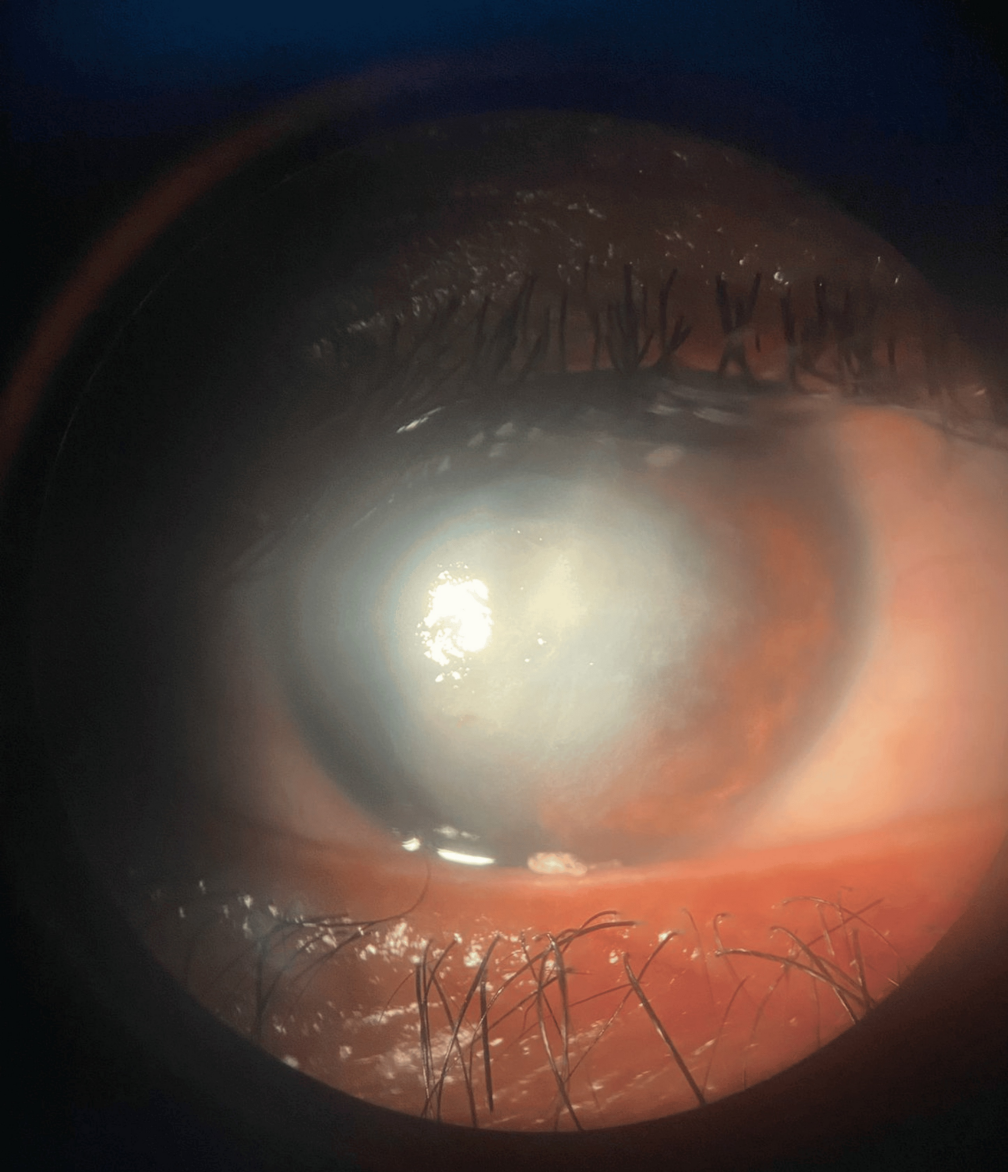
B-mode ultrasound of the RE after intrastromal instillation of voriconazole + corticosteroid therapy locally and cyclosporine RE: Right eye
